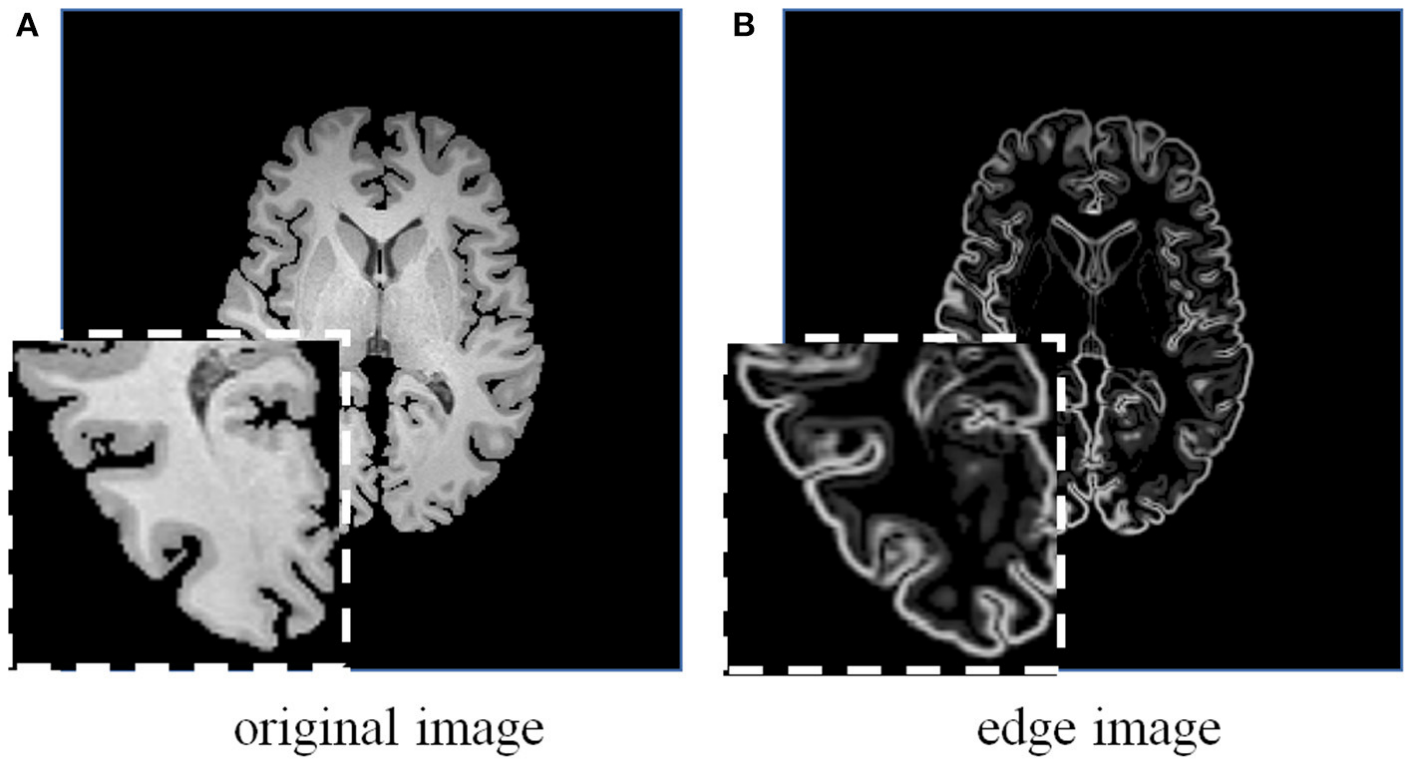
A brain MRI image (A) and its corresponding edge map (B) generated using 3D Sobel operator. The edge map can more explicitly represent the structural information.
